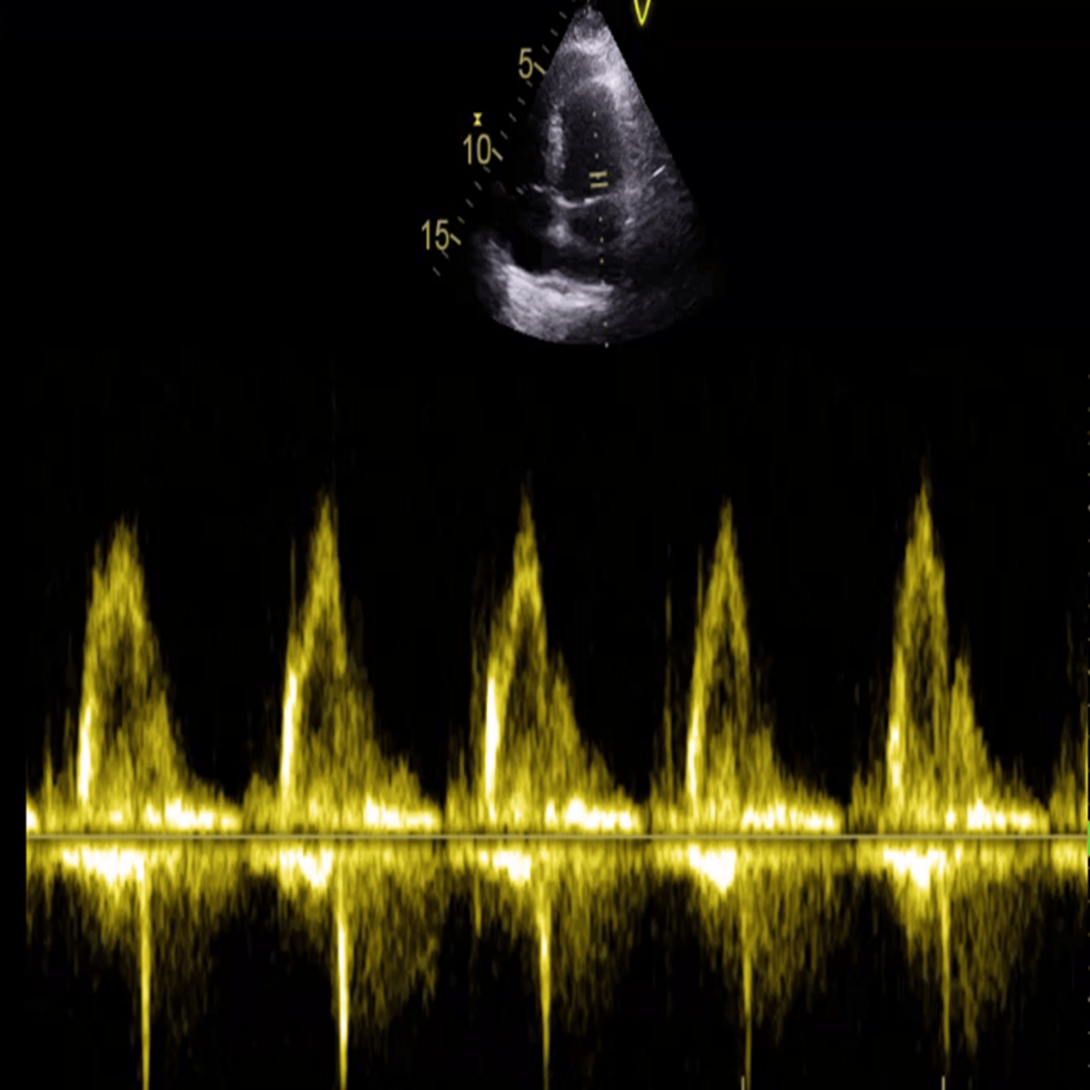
The echocardiogram showing well-defined heart chambers and properly functioning valves, with no evidence of structural abnormalities. The waveforms on Doppler imaging indicate normal blood flow and no signs of stenosis or regurgitation.
